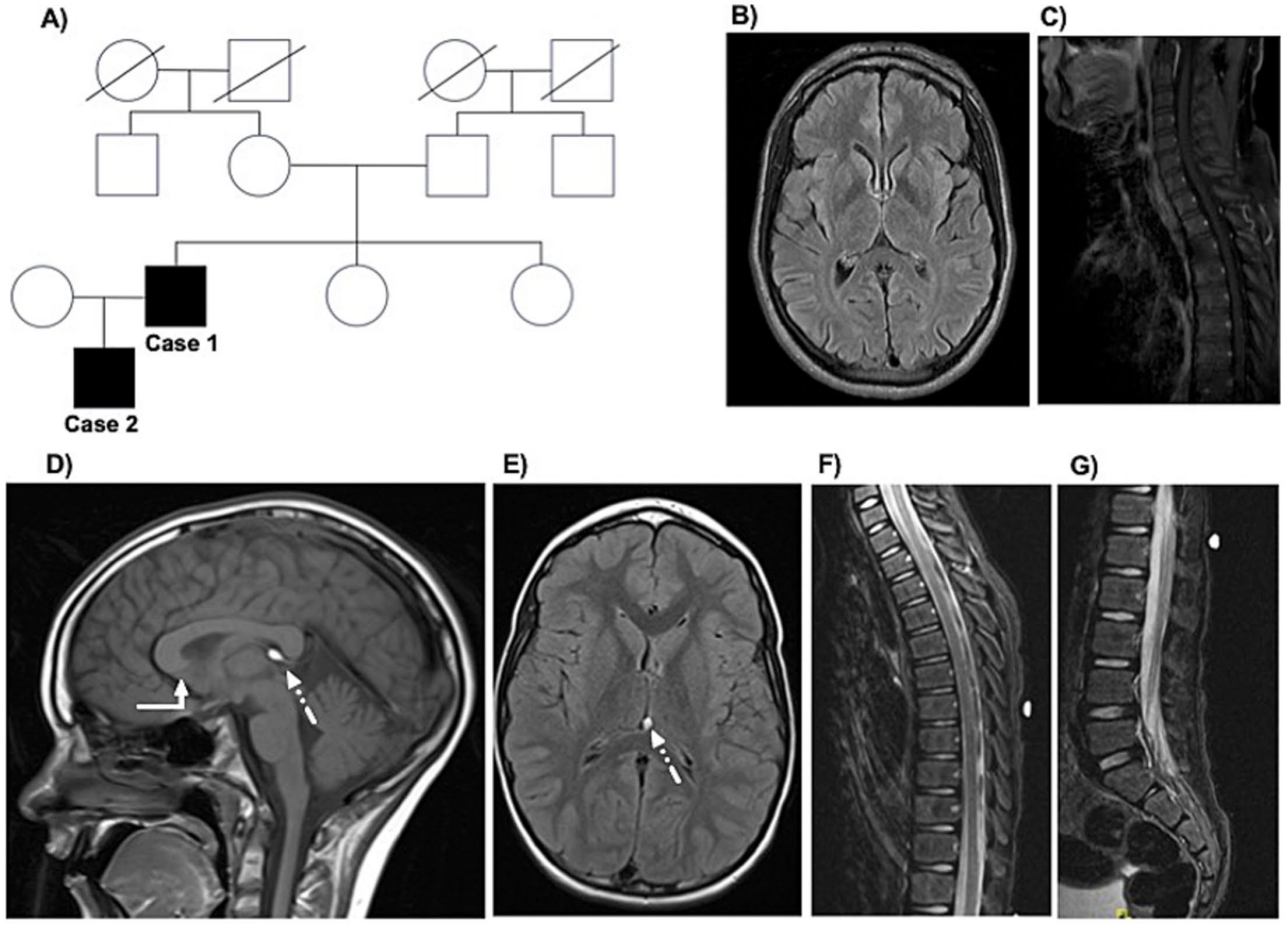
The family tree and MRI findings in the father-son cases. The family tree is displayed in panel A. Parts of the magnetic resonance imaging (MRI) of Case 1 obtained at age 44 at the initial movement disorders clinic appointment are displayed, including axial FLAIR sequences of the brain (panel B) and upper spine (panel C). Selected images of the MRI brain and spine of Case 2 obtained at age 8 at initial child neurology appointment are displayed, including sagittal T1-weighted (panel D; white arrow refers to the lack of visualization of the rostrum and mildly dysmorphic corpus callosum; dotted arrow refers to the 6-mm lipoma in the cavum vellum interpositum) and axial FLAIR sequences (panel E) of the brain and sagittal short tau inversion recovery (STIR) sequences of the upper and lumbar spine (panels F,G).
